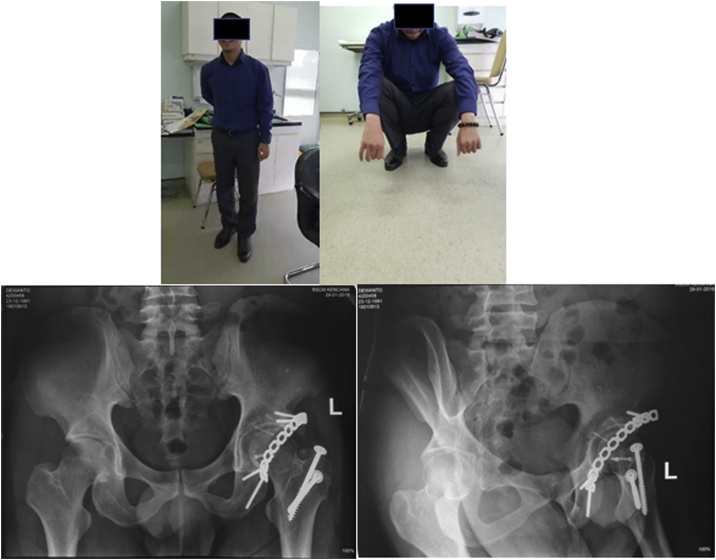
Patients were followed up for 2 years & X-ray after 2 years follow up.
